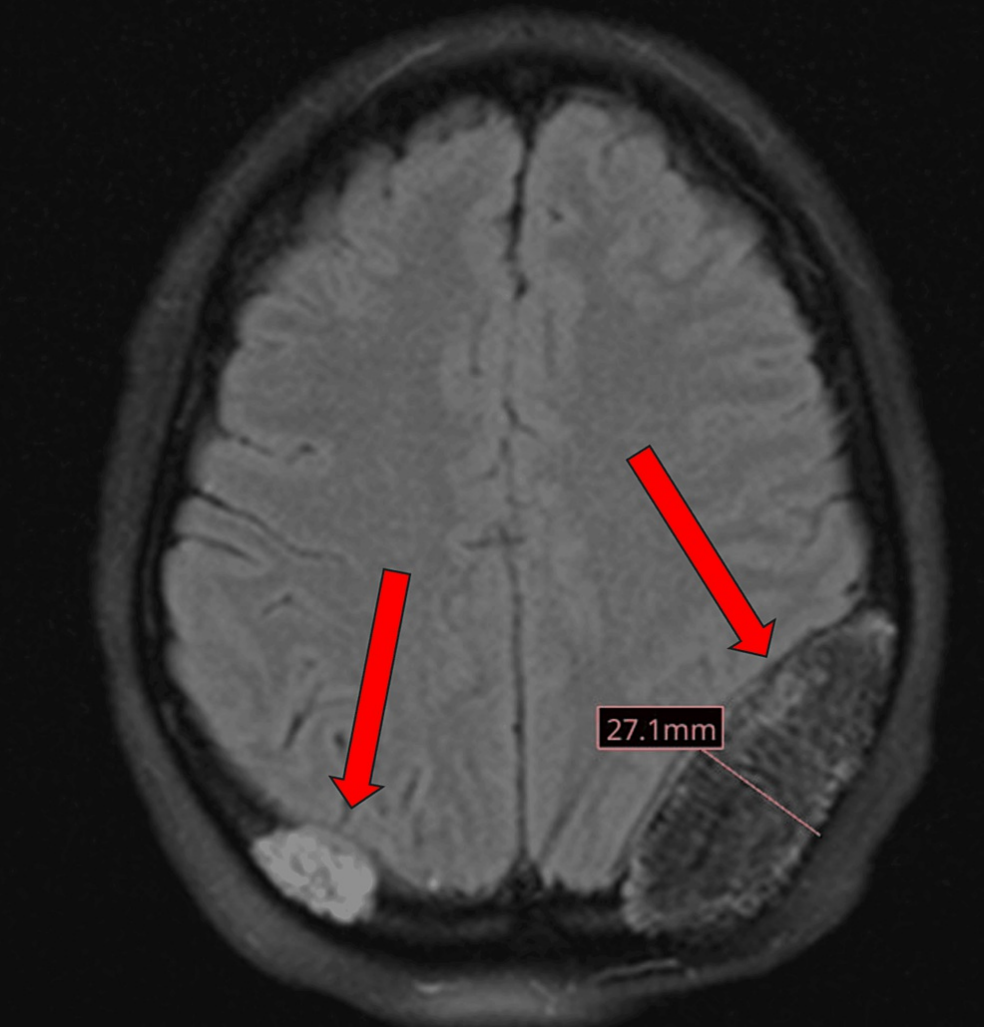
MRI brain T2 axial flair (27.1 mm) This is the initial imaging for the patient's brain masses found on MRI, suggestive of parietal bone hemangioma. MRI, magnetic resonance imaging
